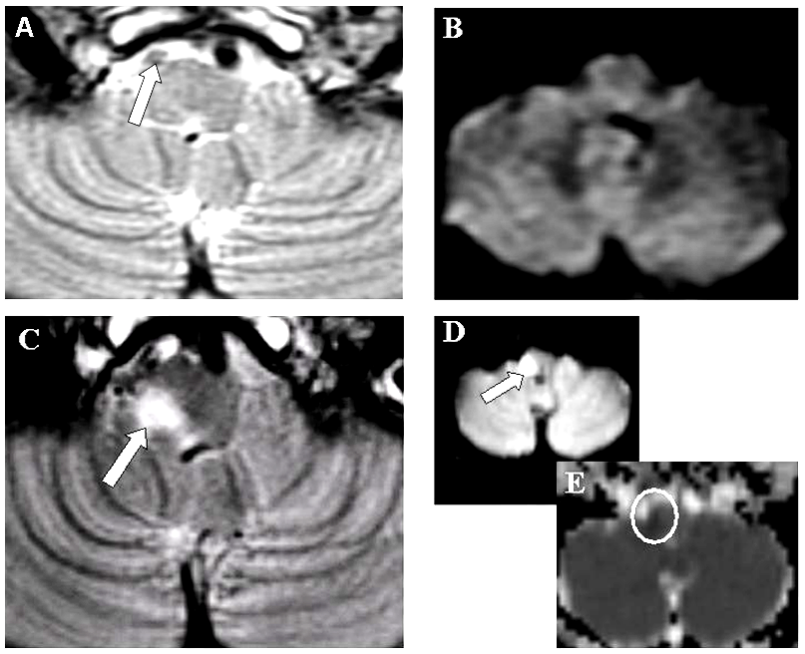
Axial T2-weighted and diffusion weighted images. A and B: Axial T2-weighted and diffusion weighted images (DWI) of the MRI 24 hours after presentation. The thin white arrow denotes the absent flow void of the right vertebral artery. C, D and E: Axial T2-weighted, DWI and apparent diffusion coefficient (ADC) of the same region 4 days later. The bold white arrows denote the infarct at the right medullary region and the white circle indicates the low ADC.
